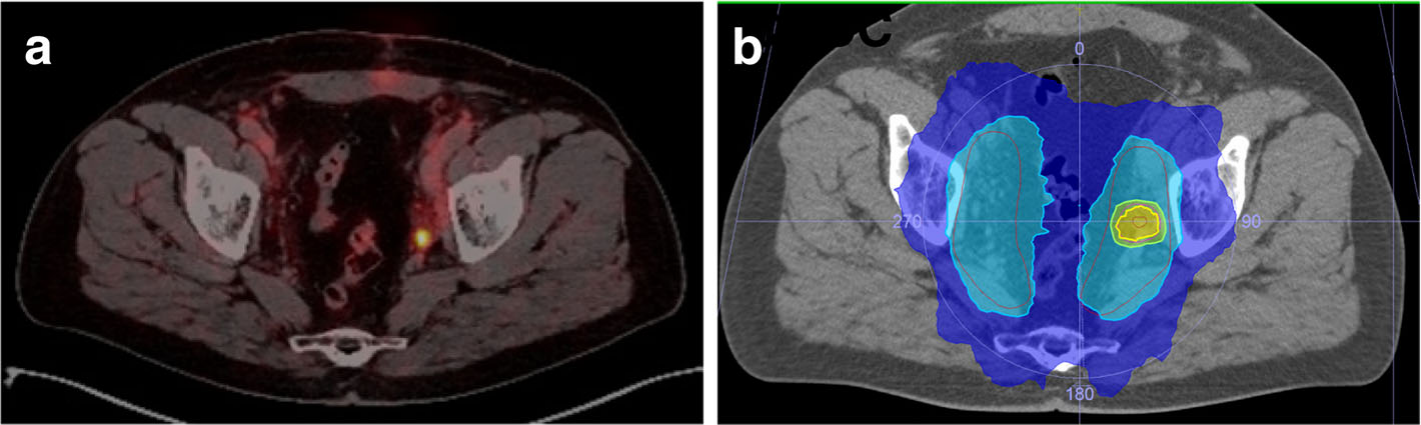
^68^Ga-PSMA PET/CT (a) and target volume with simultaneously integrated boost volume (b) to PET-positive lymph node
